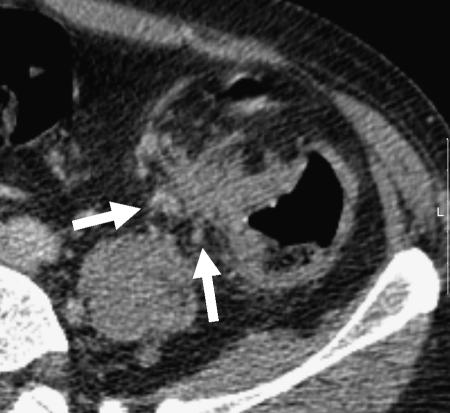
Preoperative CT scan showing a bulky tumour of the descending/sigmoid junction. There is irregular nodular extension medially (arrows) indicating T3 infiltration. This is likely to extend through the medial nonperitonealised, mesenteric surface of the colon. The pathological stage was confirmed as pT3.
